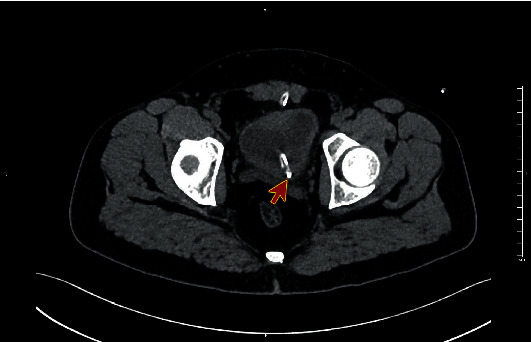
Computed tomography (CT) abdomen, axial view showing the tip of the suprapubic catheter inserted at the left ureterovesical junction (red arrow).
